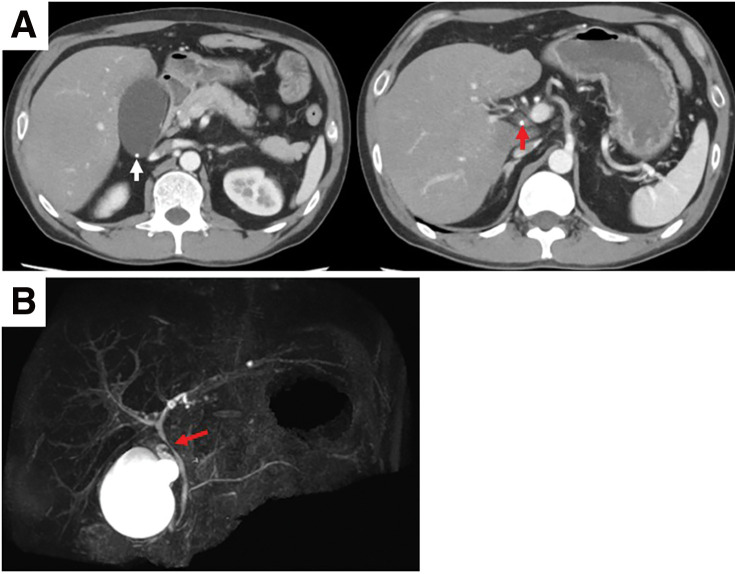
The image findings after the initial surgery. (A) Contrast-enhanced computed tomography showed an enlarged gallbladder with slightly enhancement of the gallbladder bed. The white arrow shows gallstones in the gallbladder. The red arrow shows gallstones incarceration in the cystic duct. (B) On magnetic resonance cholangiopancreatography, the biliary tract abnormality was not presented, and the common bile duct stones were not detected. The red arrow shows the gallstone pressing on the common bile duct.
